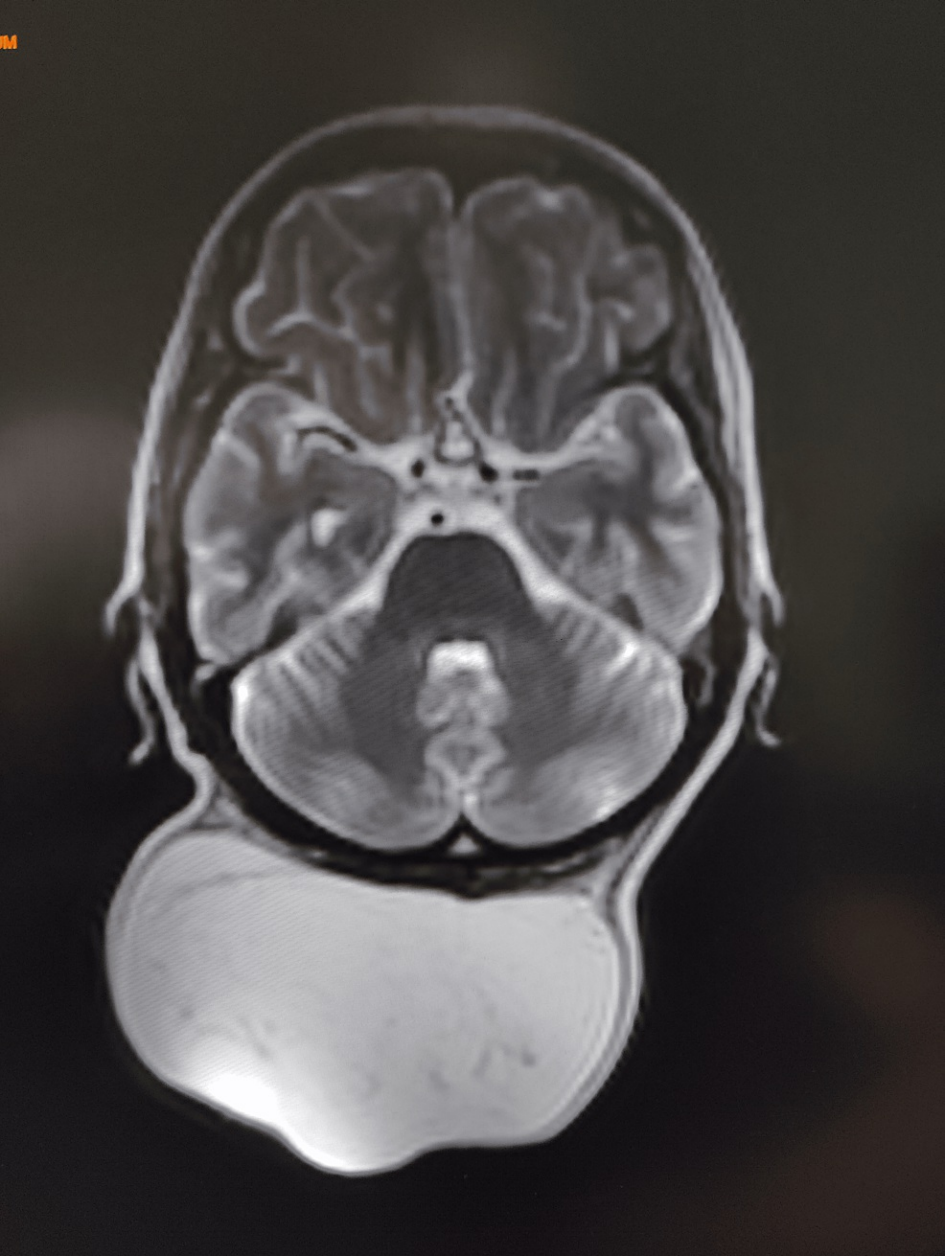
Cervical MRI image showing the giant lipoma in the cervical occipital area.
